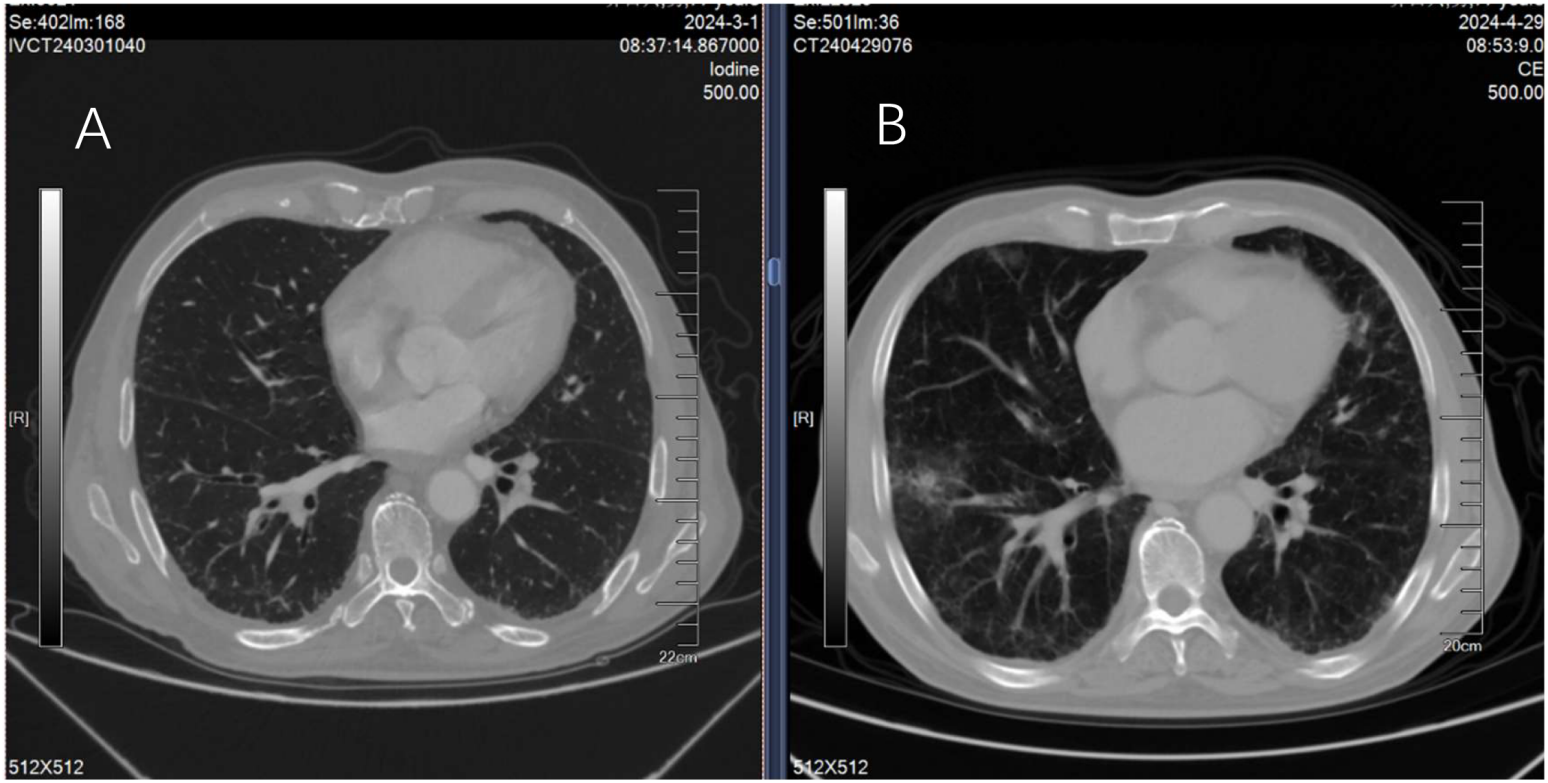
Chest HRCT examinations performed 2 months before and 1 day before nab-PTX treatment revealed no significant abnormal findings in both lungs (A, B). nab-PTX monotherapy was initiated on April 30, 2024, at a dose of 100 mg via intravenous infusion (administered on day 1 and day 8, repeated every 21 days). The patient exhibited good tolerance during chemotherapy, with no obvious adverse reactions other than significant asthenia. During the chemotherapy interval on May 4, physical examination revealed clear breath sounds over both lungs, no audible rales or rhonchi, and absence of pleural friction rub. The second cycle of nab-PTX (100 mg, intravenous infusion) was administered on May 7. The patient maintained independent ambulation during treatment, with no cough or expectoration; auxiliary examinations showed normal inflammatory markers including white blood cells and neutrophils.
